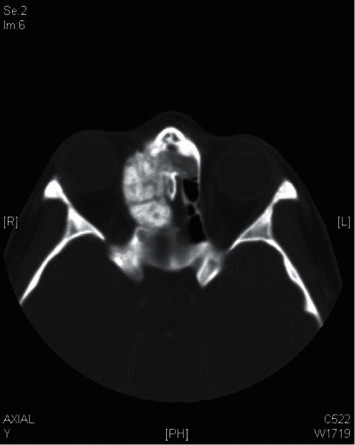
The paranasal axial CT scan demonstrates the right ethmoid sinus besides nasal hollow bulk by orbital then intracranial engrossment.
